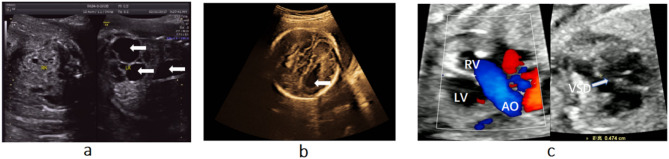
The detailed ultrasonography showing the abnormal findings in cases 1, 4 and 12, with arrows pointing to the abnormal parts. (a) Multiple anechoic areas are seen in the left kidney of case 1, suggesting polycystic kidney disease. (b) A teardrop-shaped change is observed in the lateral ventricle of case 4, indicating a possible absence of the corpus callosum. (c) In case 12, the right ventricle (RV) is connected to the aorta (AO), suggesting double outlet right ventricle, and a ventricular septal defect measuring 0.474 cm is present
